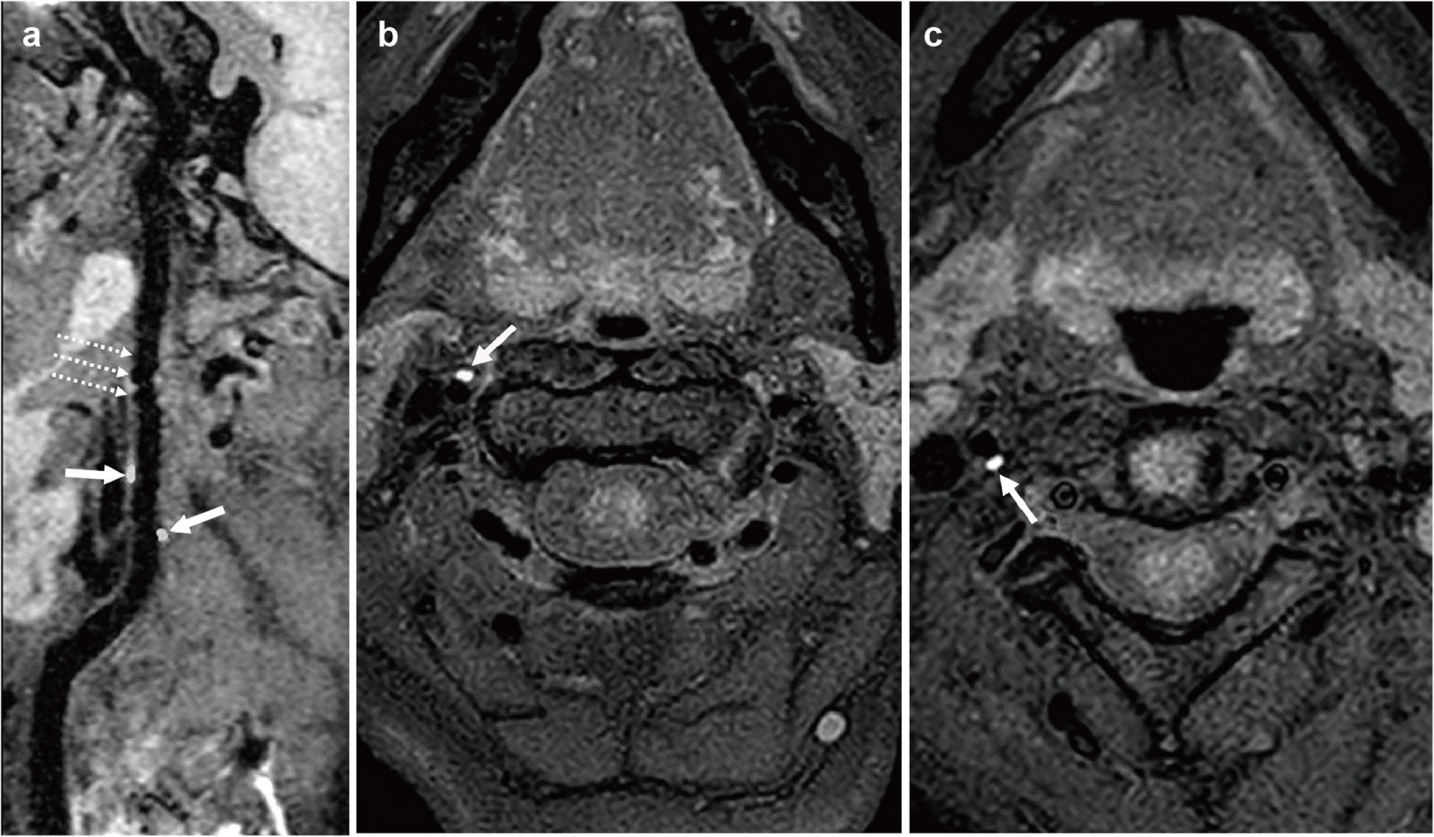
3-month follow-up imaging. a High resolution magnetic resonance imaging of the vascular wall (black-blood T1WI) showed the "string-of-beads” appearance (white dotted line arrow) and two hyperintense residual intravascular haematomas (white arrow) in the vascular wall of the right ICA; b and c. Axial imaging showed a hyperintense residual intravascular haematoma of the right ICA.
